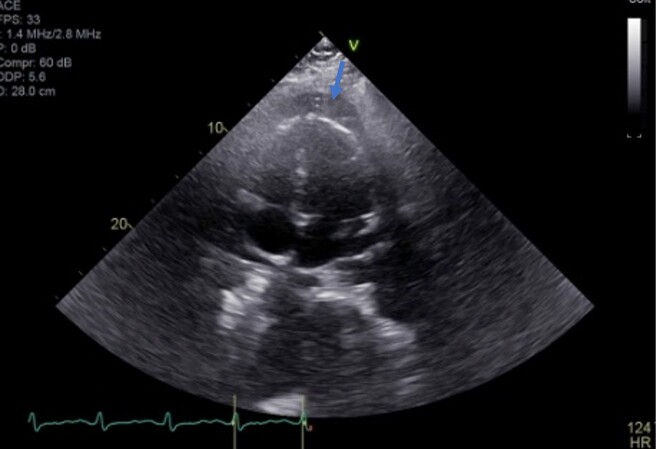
Transthoracic echocardiogram four-chamber view demonstrating pericardial effusion (arrow).
